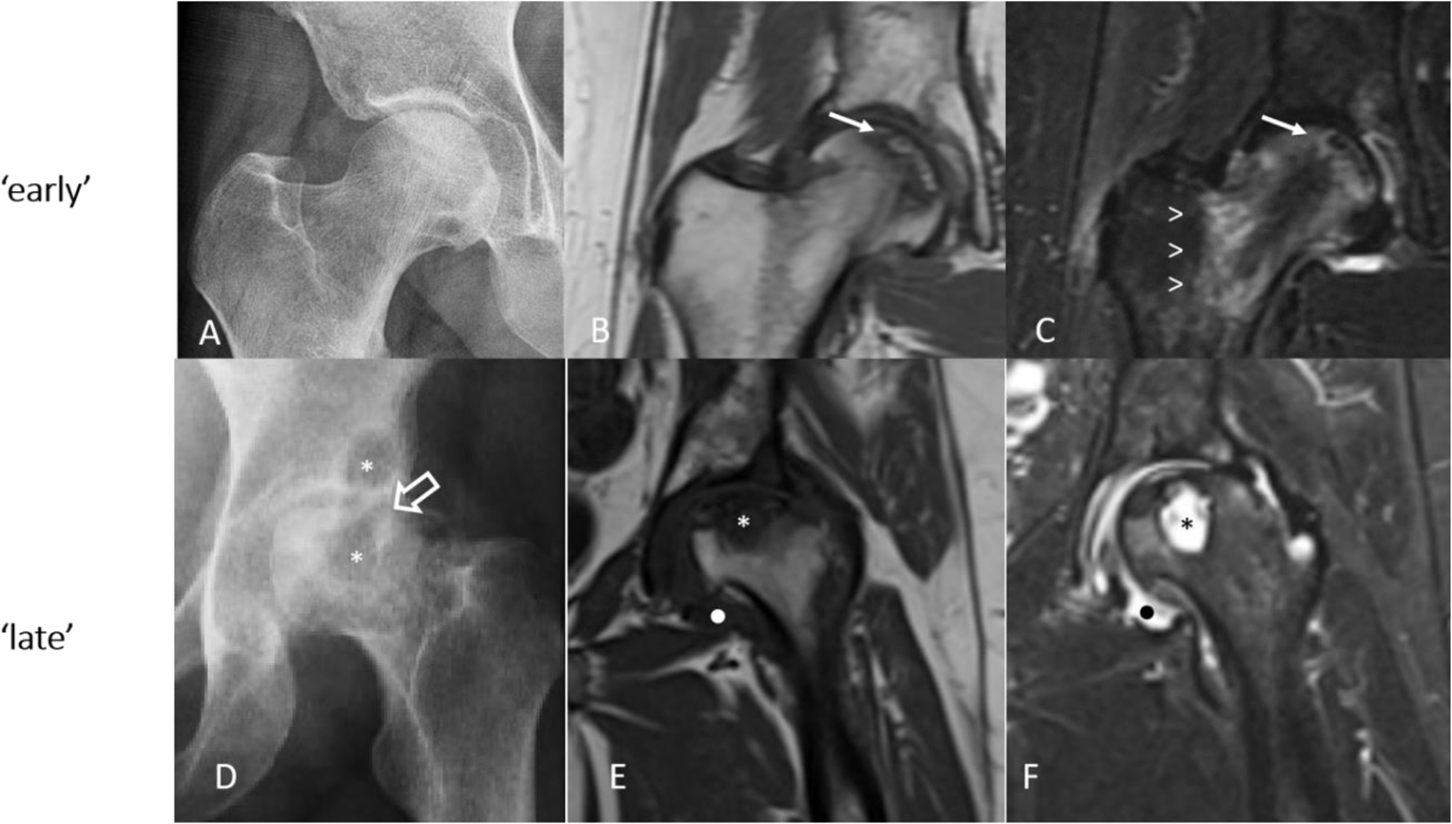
‘Early’ hip osteonecrosis in a SLE patient (A-C). Conventional radiography A shows mild and initial signs of osteoarthritis (i.e., subchondral sclerosis of the femoral head). Coronal magnetic resonance imaging (T1-weighted, B; STIR, C) shows the characteristic ‘double line’ sign (arrow) and the presence of diffuse bone marrow edema (arrowheads). ‘Late’ hip osteonecrosis in a SLE patient (D-F). Conventional radiography (D) shows osteochondral collapse (open arrow) and irregularity of the femoral head. There is cystic change within the femoral head and subchondral cyst formation within the lateral acetabulum (asterisks). Magnetic resonance imaging (T1-weighted, E; STIR, F) confirms the subchondral collapse in the weightbearing femoral head with a large area of subchondral damage. Note the presence of cystic change within the femoral head (asterisks) and secondary synovitis of the hip joint (rounded dots)
